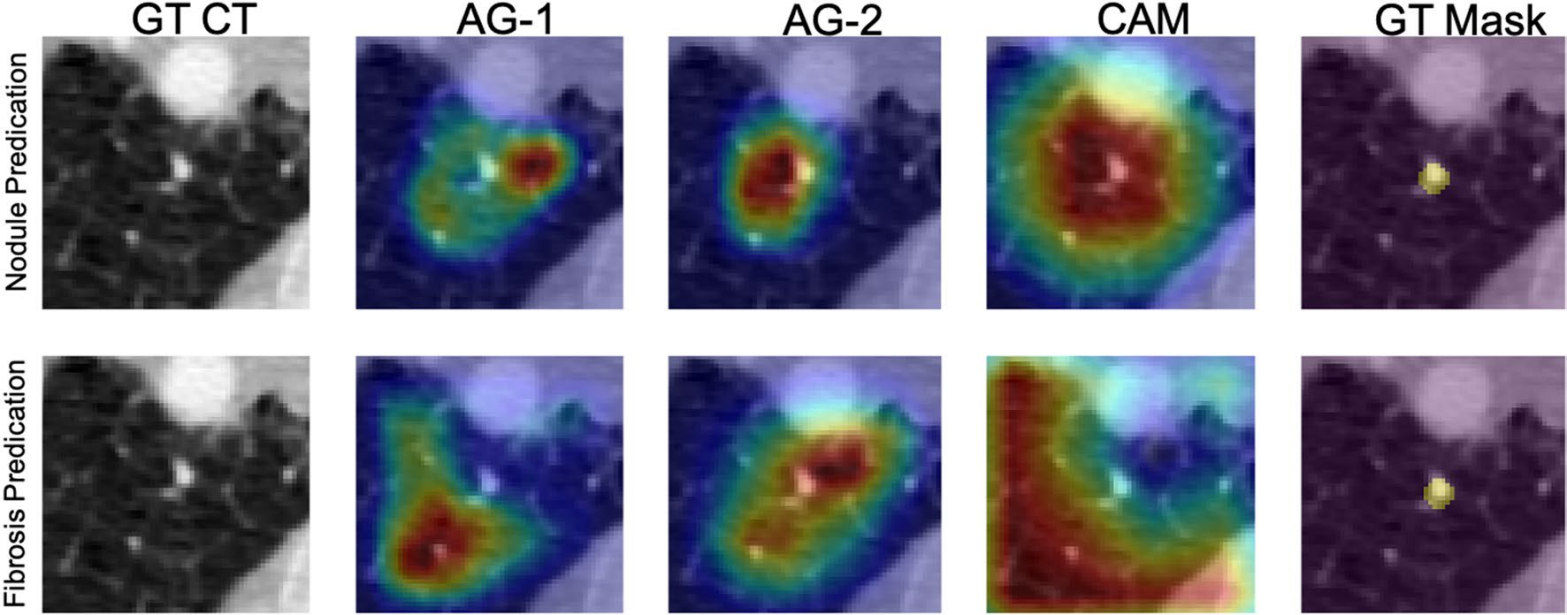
Network attention gates (AGs) and class activation maps (CAMs) visualizations. An example is network visualization for nodule prediction (first row) and lung fibrosis prediction (second row) tasks. The first, second, third, and fourth columns indicate the ground-truth (GT) CT image, first attention gate (AG-1) at No. 11 layer depth, second attention gate (AG-2) at No. 14 layer depth, and the class activation map (CAM) at the final layer, respectively. The fifth column indicates the nodule ground-truth mask (GT Mask), which is not available when the model was trained. The case demonstrated here is a benign nodule in the non-fibrotic lung, where both nodule malignancy and fibrosis models made the correct inferences. From the AGs and CAMs, we can observe the nodule network focuses on nodule parenchyma and its surrounding tissues, while the fibrosis network focuses on other lung tissue with the nodule parenchyma excluded
